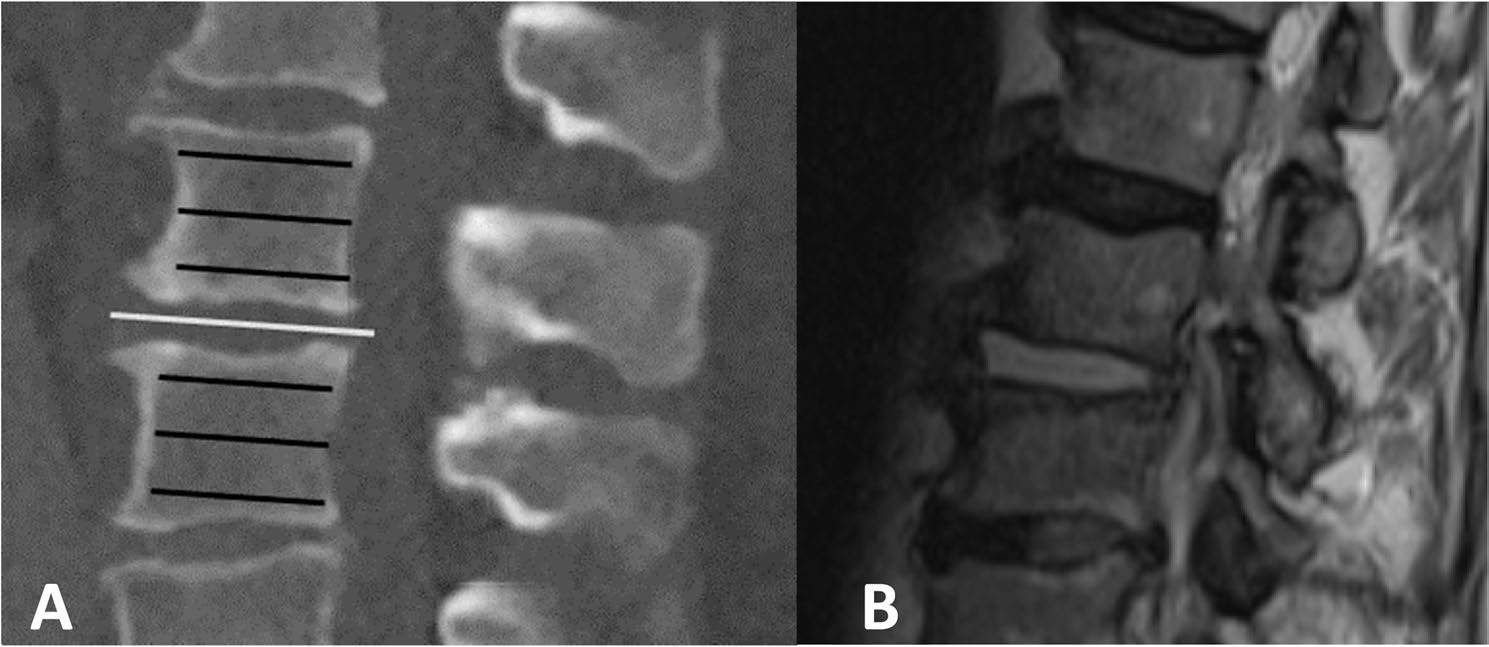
Localization of the performed measurements. A shows a lateral reconstruction of a lumbar spine CT Scan. In the vailable T2 MRI Sequence (B) of this patient the intervertebral disc between L2 and L3 showed inflammatory changes. This disc was classified in the CT (A) as an affected disc (white bar). The intervertebral disc one segment above and one segment below served as reference. The black bars show the localization of the performed measurements in the vertebral bodies which were affected by the spondylodiscitis. The unaffected adjacent vertebral bodies from the level above and below as reference
